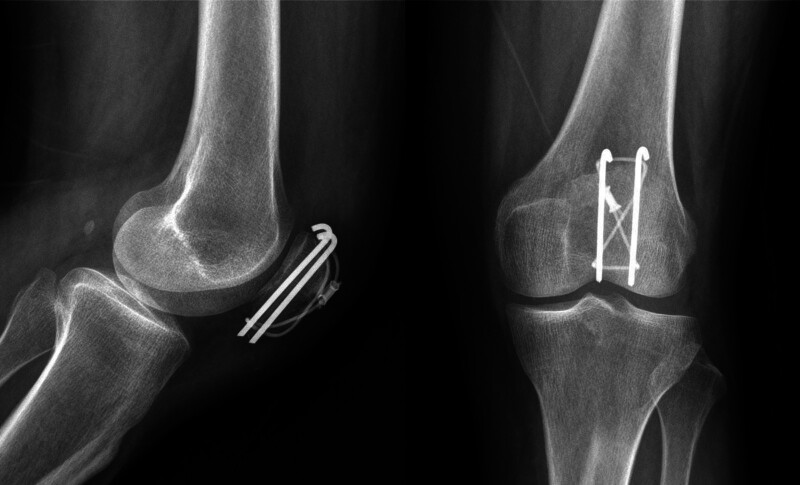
The 1 month postoperative X-ray film showed satisfactory reduction and fixation of the patellar fracture.
